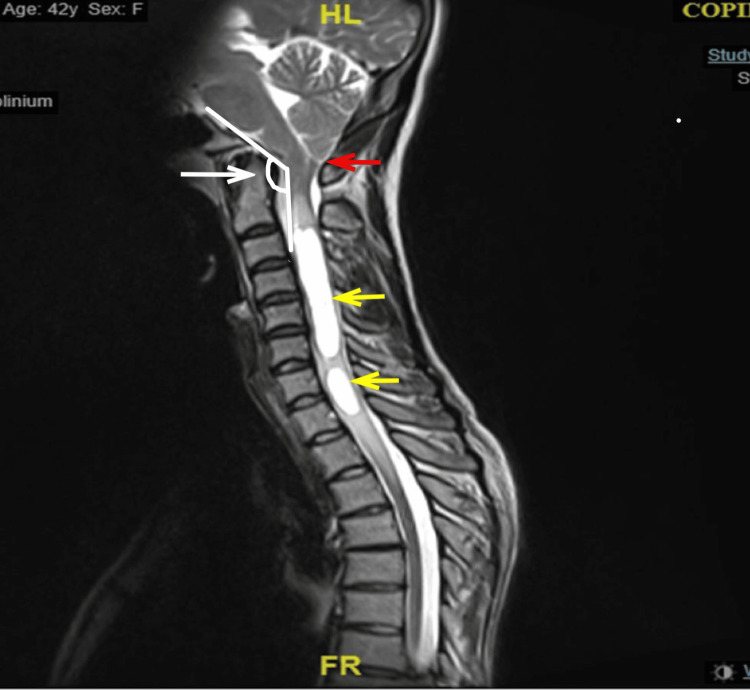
MRI of the patient showing cerebellar tonsillar herniation and distortion of the medulla highlighted by the red arrow. Two high-intensity lesions, highlighted by yellow arrows, are seen largely within the cervical cord. The clival canal angle is denoted by the white arrow.
